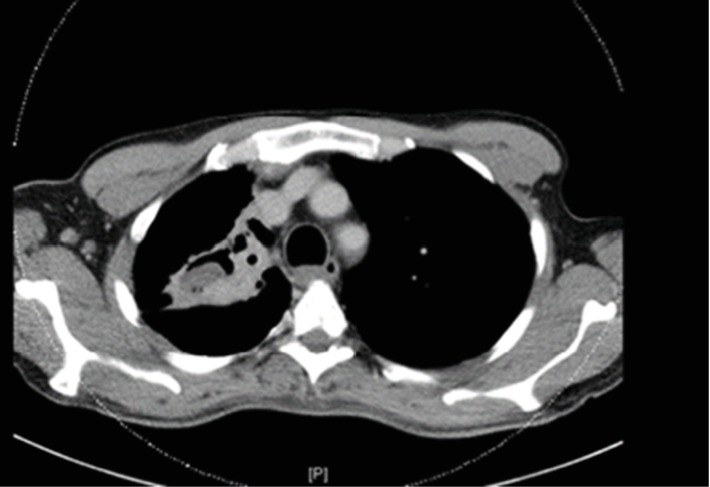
CT showing cavitation and partial collapse of the right upper lobe.
